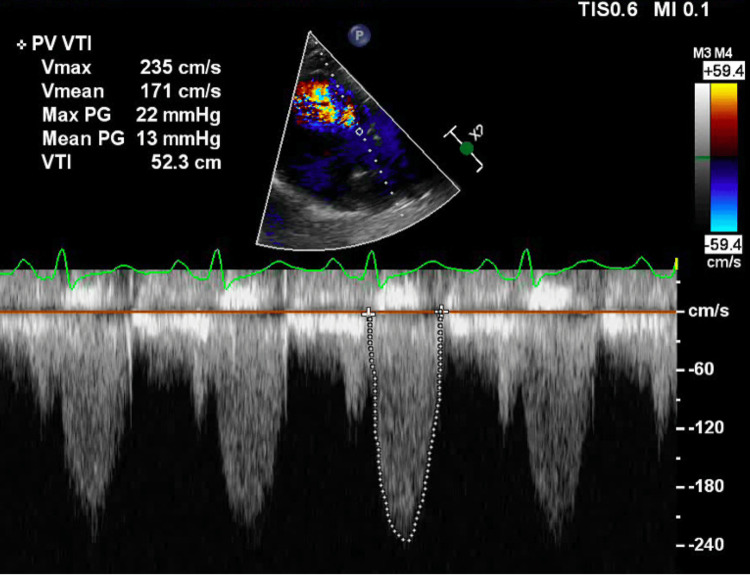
Parasternal short-axis of the right ventricular outflow tract, showing mild pulmonary stenosis.
